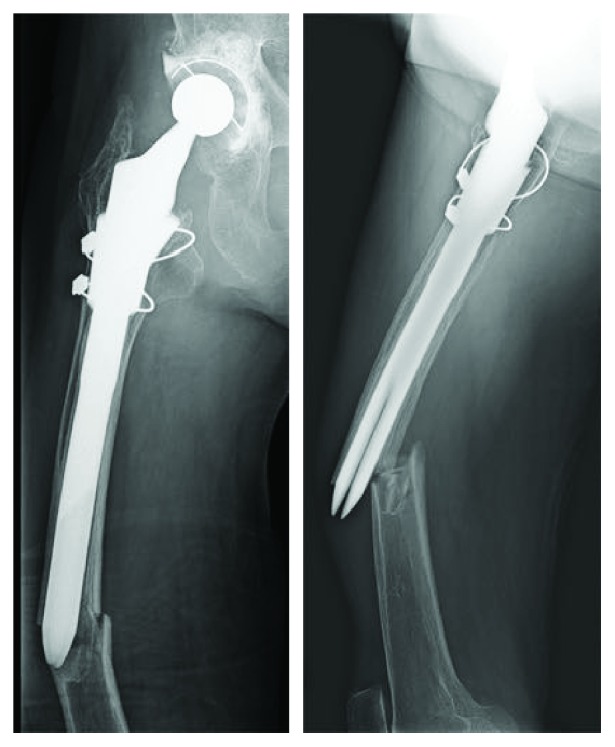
Radiograph showing a noncomminuted transverse fracture located at the tip of the well-fixed femoral stem with a medial spike, third bone fragment.
